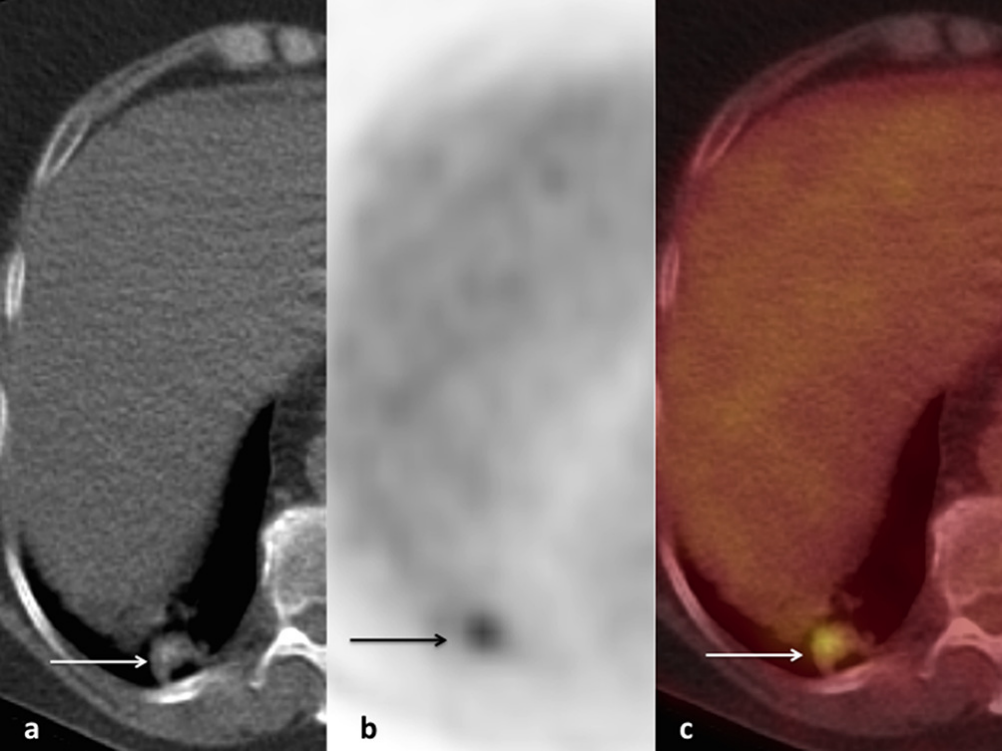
Axial non-contrast CT scan (a), FDG-PET scan (b), and fused PET/CT scan (c) showing focal increased FDG uptake within the right lower lobe nodule (arrows), maximum standardized uptake value of 5. FDG, ^18^F-fludeoxyglucose; PET, positron emission tomography.
